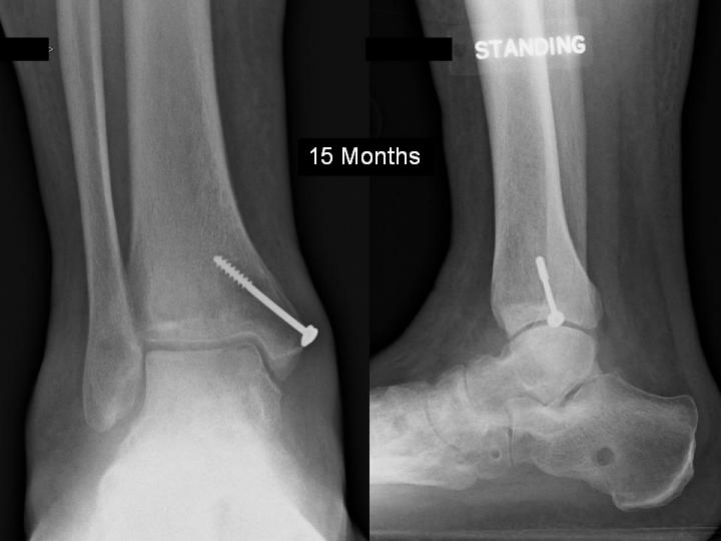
Anterio-posterior and lateral radiograph at 15 months.
